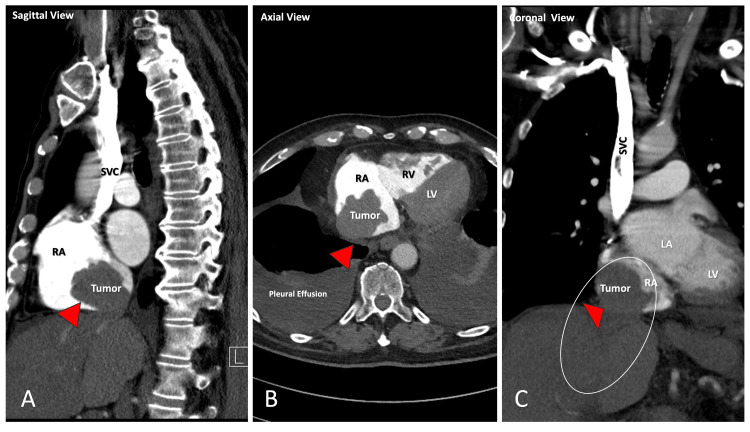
Initial chest CT angiography (CTA) imaging demonstrating a large lobulated filling defect in the RA suspicious for malignancy or tumor thrombus in (A) sagittal, (B) axial, and (C) coronal views IVC: inferior vena cava; SVC: superior vena cava; RA: right atrium; RV: right ventricle; LA: left atrium; LV: left ventricle
